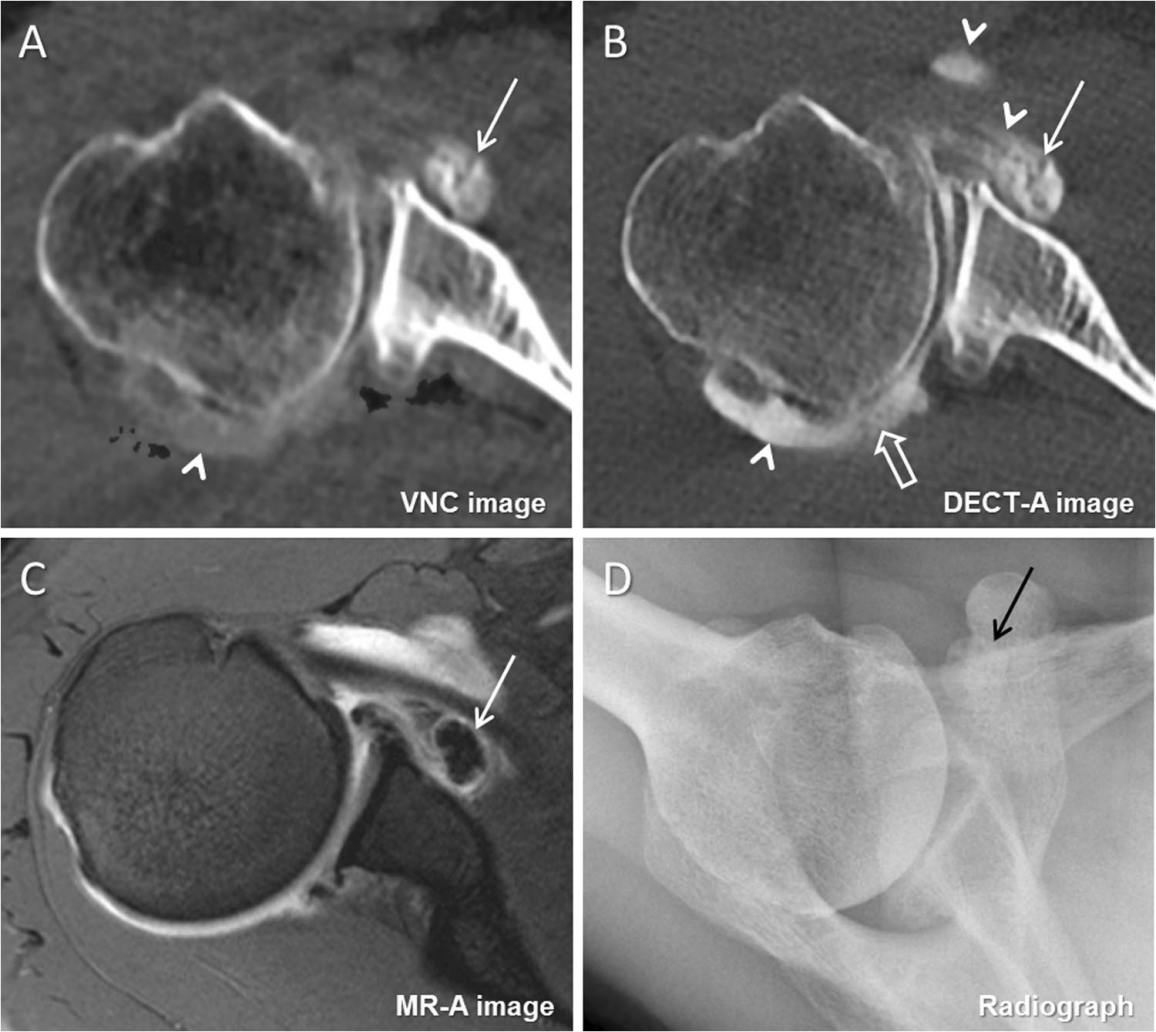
A 37-year-old male with dysplasia of the posterior glenoid and osteoarthritis. On the axial virtual non-contrast image (A), a large ossified intraarticular loose body is visible in the subscapular recess of the glenohumeral joint (arrow). On the axial DECT arthrography image (B), the intraarticular loose body (arrow) was missed because of similar density as the adjacent iodinated contrast material (arrowheads). The loose body was interpreted as synovitis of the subscapular recess as it is the case for the posterior recess (open arrow). The presence of the intraarticular loose body (arrow) was confirmed on the axial MR arthrography image (C) and on the axial radiograph (D). Note the incomplete subtraction of iodine (arrowhead) in the posterior recess on image (A)
